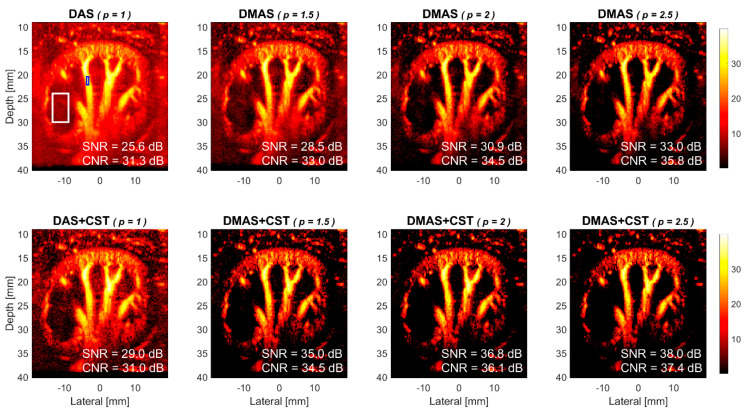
Experimental power Doppler images of rabbit’s kidney for DAS beamforming, DMAS beamforming with the *p* value of 1.5, 2.0, and 2.5, respectively from left to right. Six PW transmit angles are used (i.e., [−5° −3° −1° +1° +3° +5°]). Upper panels: without the CST technique. Lower panels: with the CST technique.
